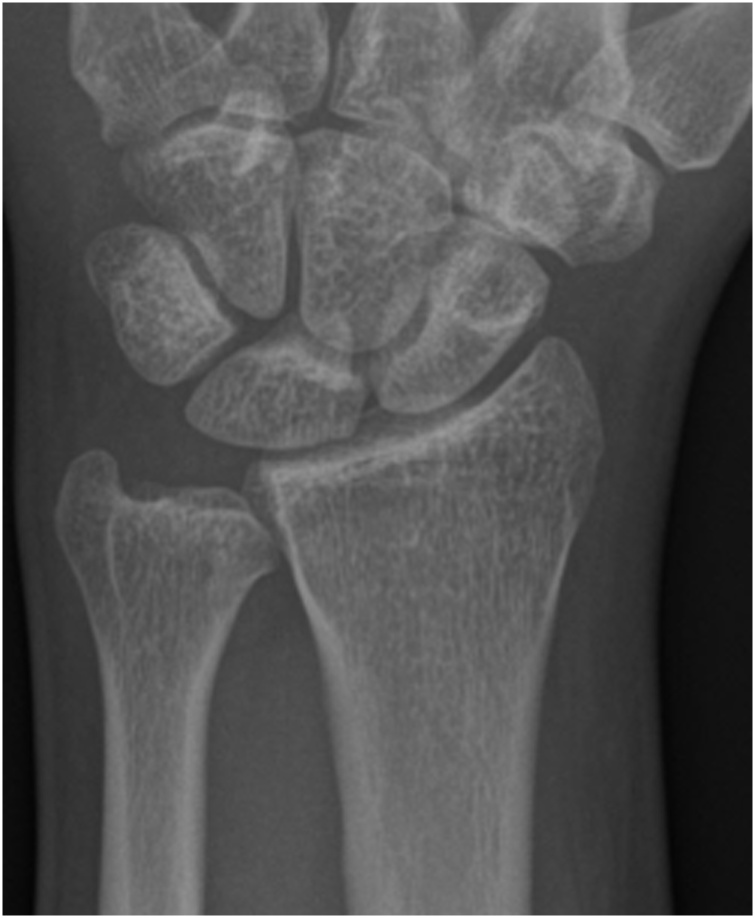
Initial AP radiography of the patient`s left wrist.
